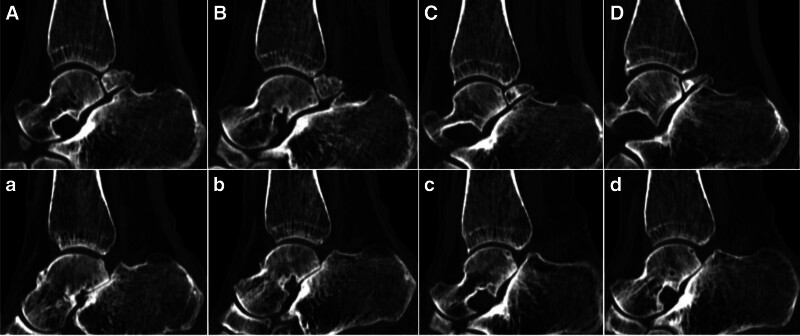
(A–D) Preoperative sagittal CT scan radiograph showing a bipartite talus with concomitant degenerative changes of the subtalar joint. (a–d) Preoperative sagittal CT scan radiograph showing the basic removal of the bipartite talus. CT = computed tomography.
